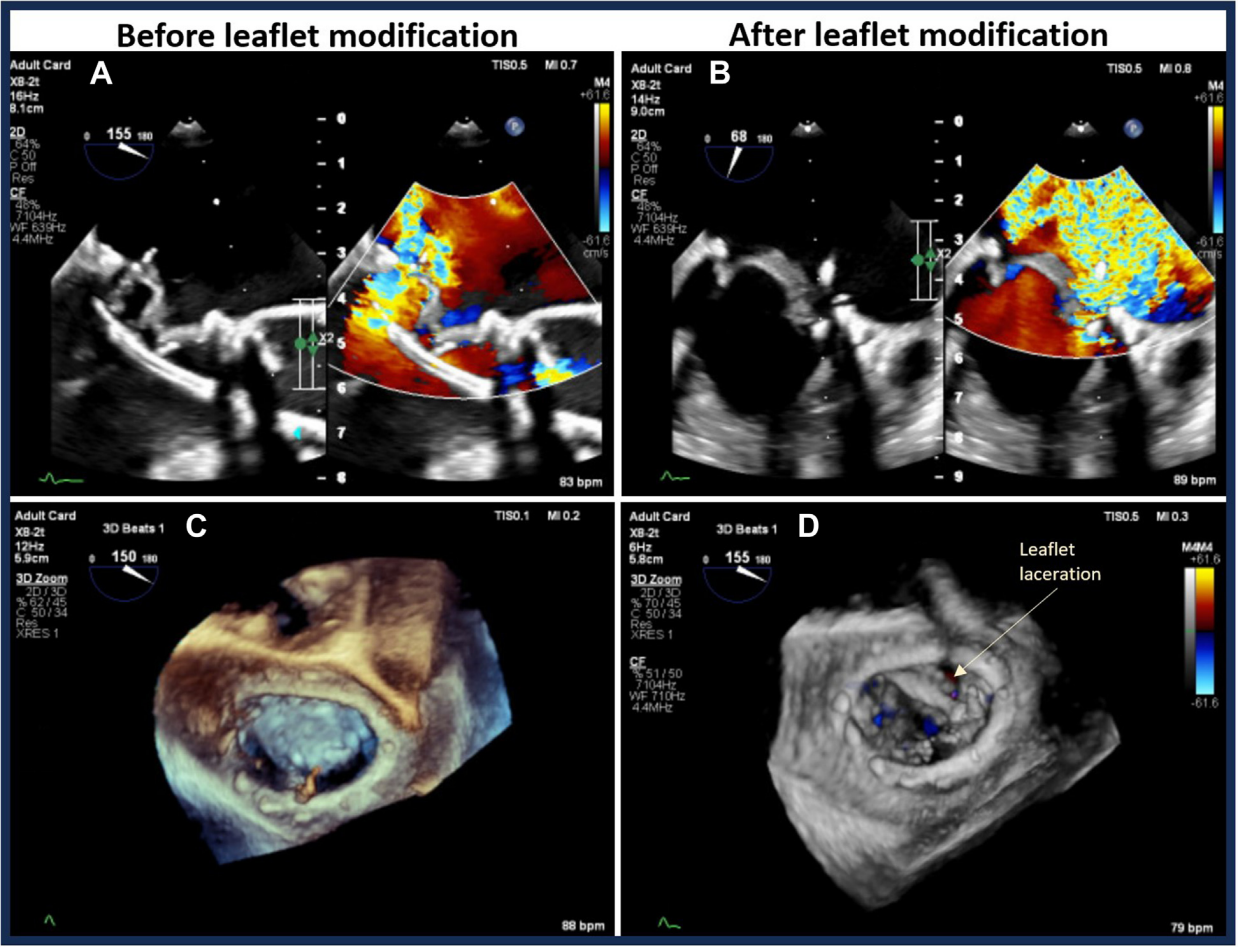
Anterior Mitral Valve Leaflet Laceration Before (A and C) and after (B and D) modification. (A) The “flying V” configuration of catheters and wire. (B) The impact of leaflet laceration with significant worsening of mitral regurgitation on color Doppler. (C and D) The appearances on 3-dimensional transesophageal echocardiography before (C) and after (D) leaflet laceration.
